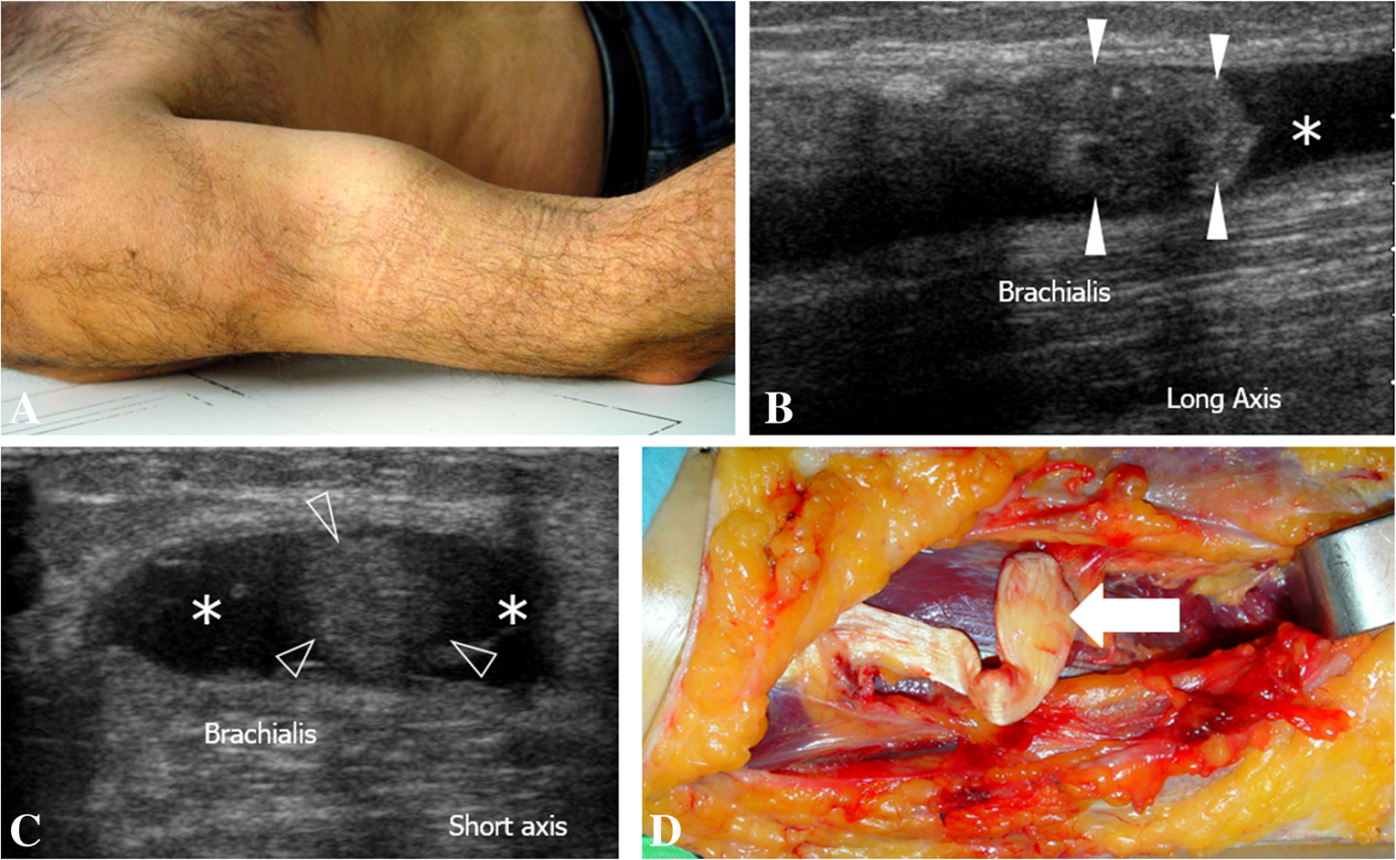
Ultrasound view of a type 3B injury (complete retracted tear due to associated EBA tear) and images of surgical confirmation. a During the physical examination, a clear tumefaction corresponding to the retracted DBBT and muscle (also known as the Popeye sign) is observed at the anterior aspect of the arm. b In the long-axis view, the DBBT stump (*white arrowheads*) presents a wavy aspect. A haematoma (*asterisk*) is observed distal to the DBBT stump. c Short-axis view of b. Cross-section of the DBBT (*arrowheads*) surrounded by a haematoma (*asterisk*). d Surgical confirmation image of b and c. The DBBT and major muscle retraction are verified. The wavy pattern of the DBBT stump (*white arrow*) correlates with that of ultrasound in image b
